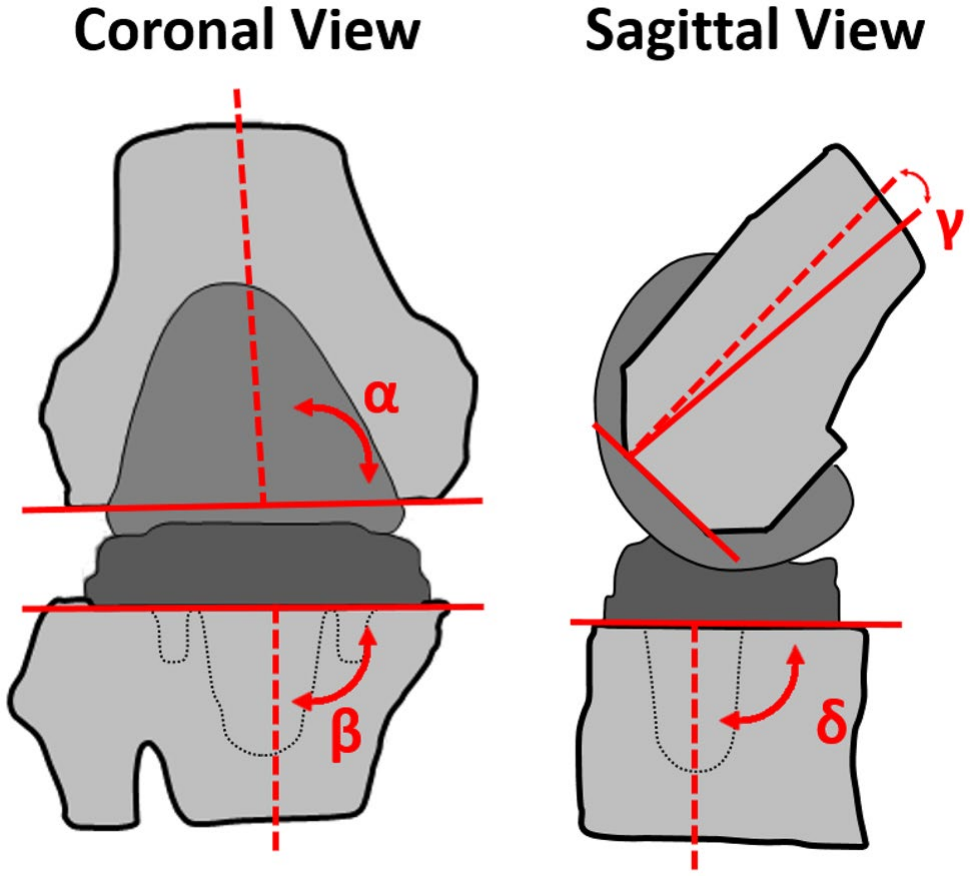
Radiographic measures of implants positioning. Coronal view. α: Femoral angle (°). β: Tibial angle (°). Sagittal view. γ: Femoral angle (°). δ: Tibial angle (°). Dashed lines represent the anatomical axes of the segments and solid lines represent the implant axes
